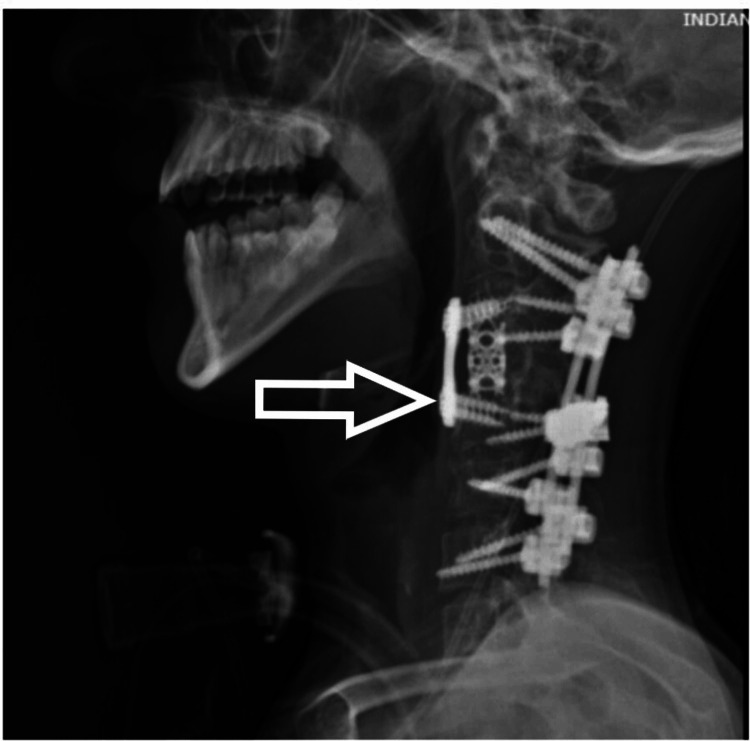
Postoperative X-ray - image 2
